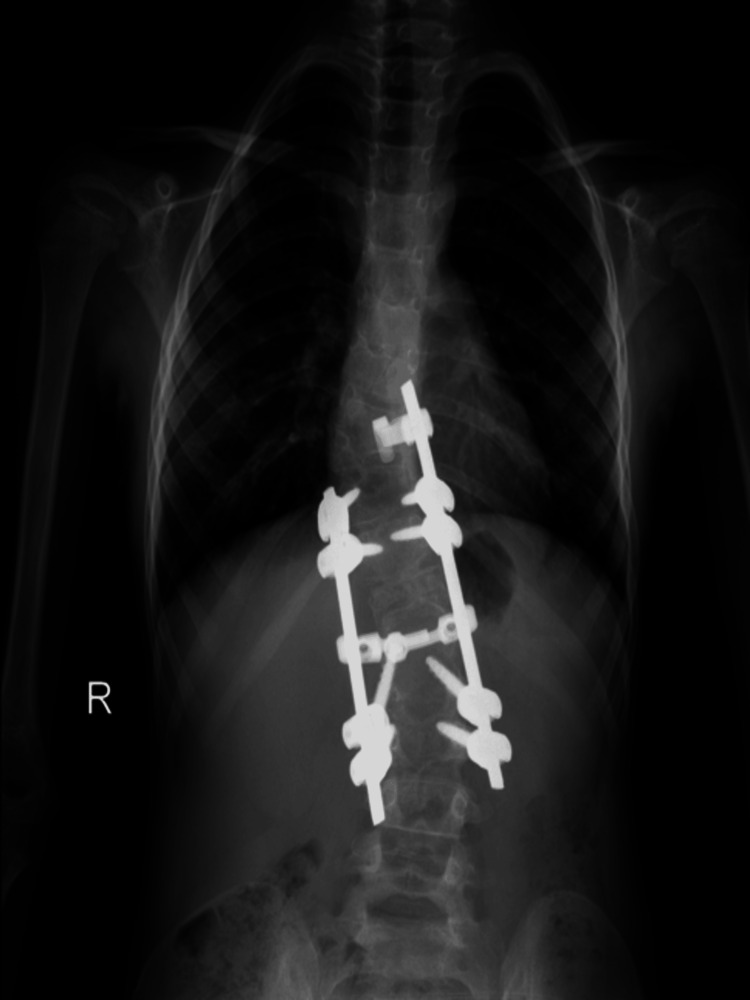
A post-operative anteroposterior radiograph of the thoracolumbar spine depicts the T10-L2 spinal fusion with pedicle screws, the T9 hook, and the resection of T12.
